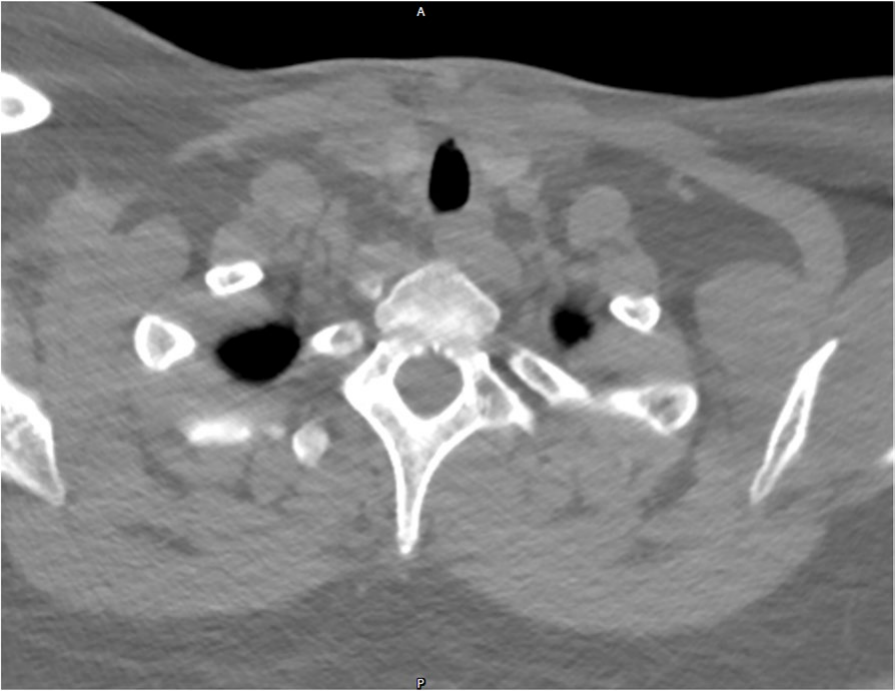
Cervical computed tomography results. After two weeks of hospitalization, showing tracheal intubation was successfully removed and tracheostomy healed without the need for further reconstruction surgery
